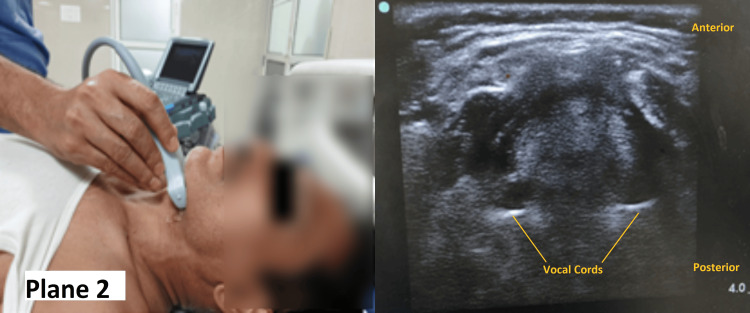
Measurement of the STT-VC Note the position of the ultrasound probe on the neck (Plane 2) and the corresponding image STT-VC: soft tissue thickness at the level of the vocal cord
